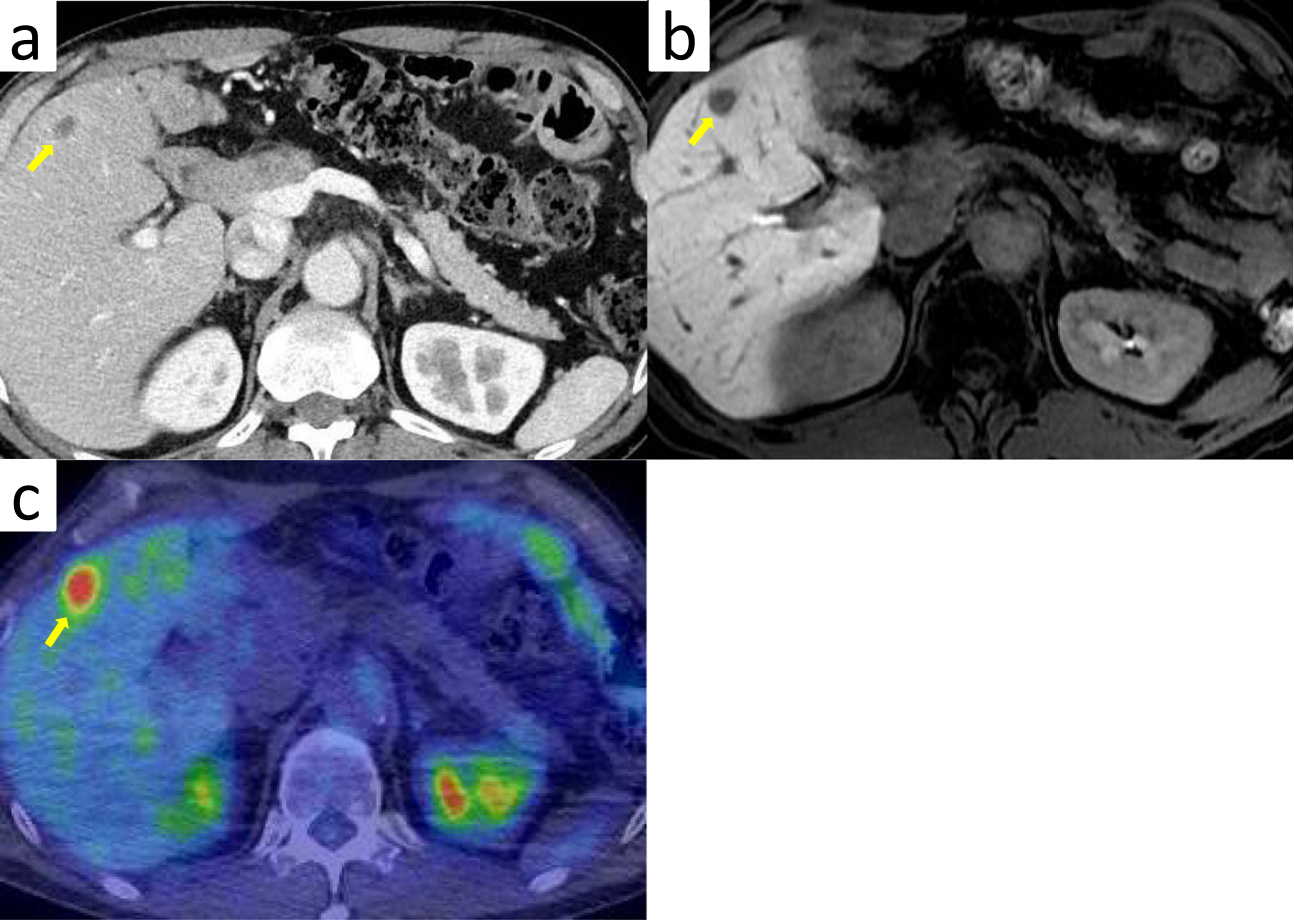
a Contrast-enhanced CT showing a 10-mm low-density area in liver segment 5 (arrow). b Gd-EOB-DPTA–enhanced MRI showing a well-defined mass in liver segment 5 (arrow). c PET/CT showing the liver mass with high fluorine-18-fluorodeoxyglucose uptake (arrow). CT, computed tomography; Gd-EOB-DPTA, gadolinium-ethoxybenzyl-diethylenetriamine; MRI, magnetic resonance imaging; PET, positron emission tomography
